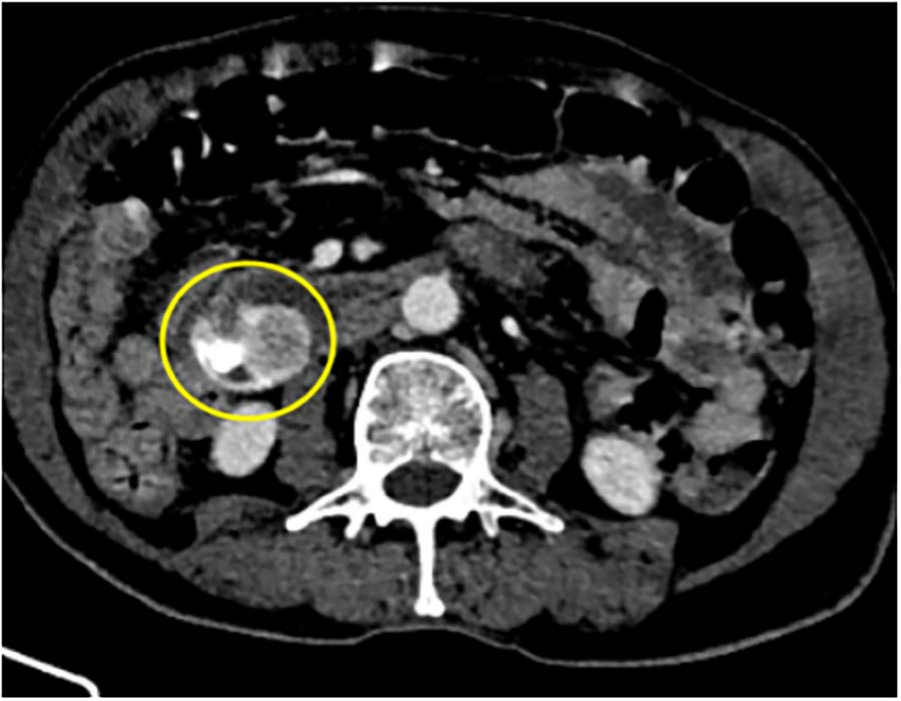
88Y/M with 2 episodes of black tarry stool, axial view of the contrast-enhanced computed tomography (CECT) abdomen showing a heterogenous mass with extravasation of contrast material, signifying the source of hemorrhage.
